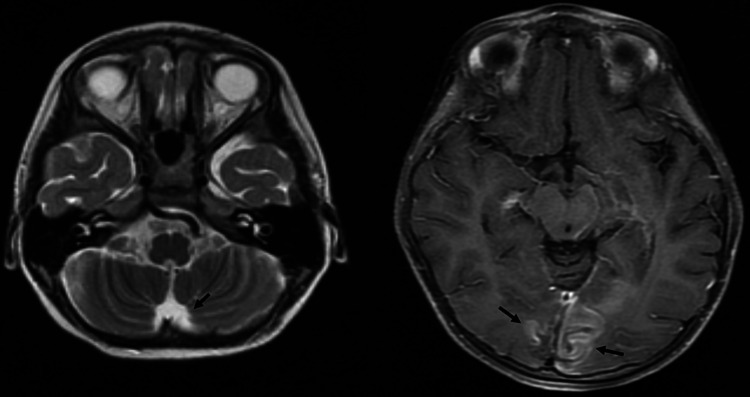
MRI of the brain at the level of the occipital lobes with T1-weighted imaging (left) and T2-weighted imaging (right), demonstrating nonspecific abnormal enhancement involving the bilateral occipital lobes without pronounced associated FLAIR abnormality.
